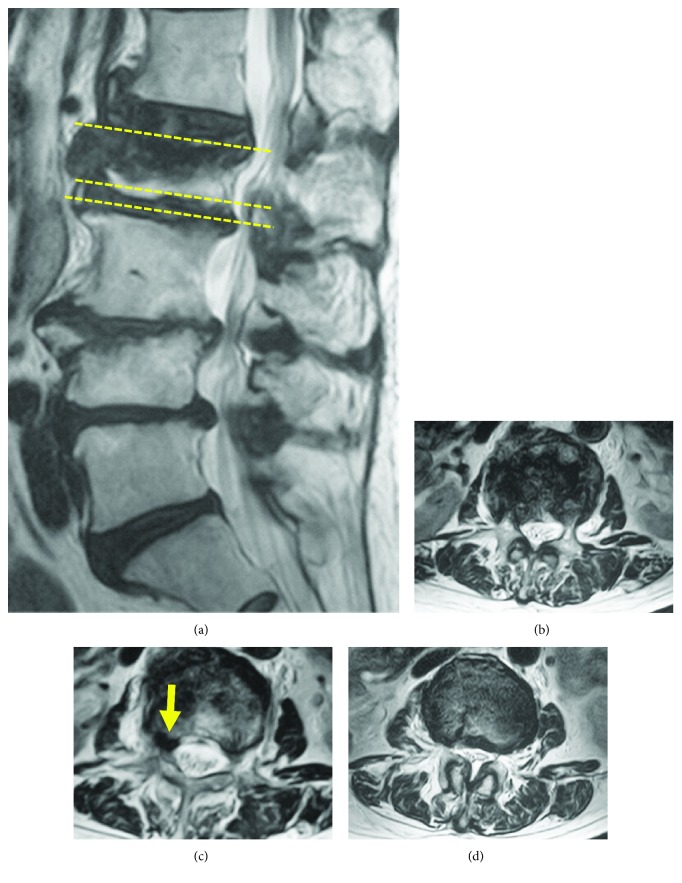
(a) Preoperative sagittal MR image showing low intensity zone in upper L2 vertebral body. (b), (c), and (d): mild canal stenosis at the L2 level and moderate stenosis at the L2/3 level. There was a mass in the rt L2/3 foramen (arrow in (c)).
